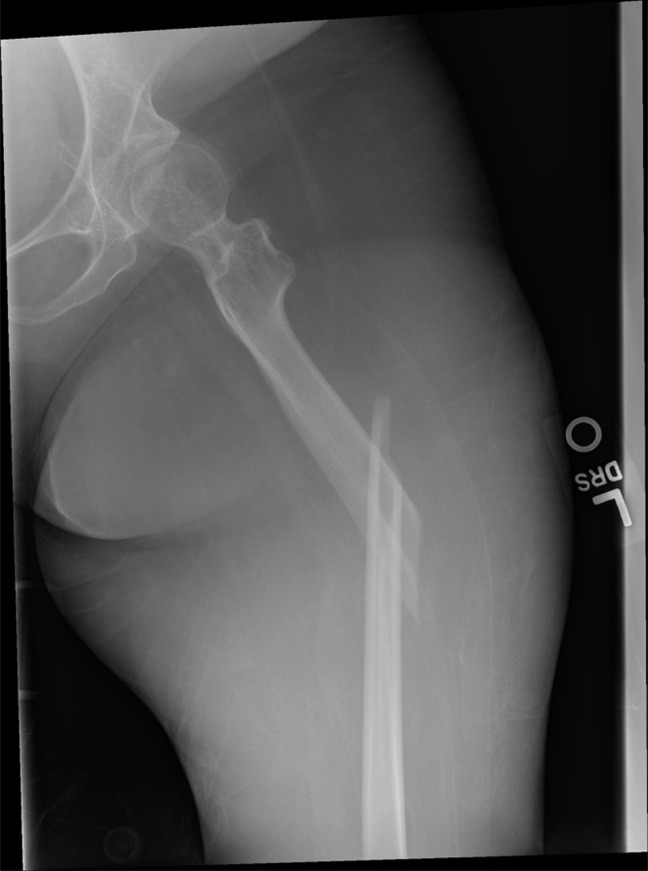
Preoperative radiographs demonstrating midshaft femur fracture in patient with miniscule diaphyseal size.
